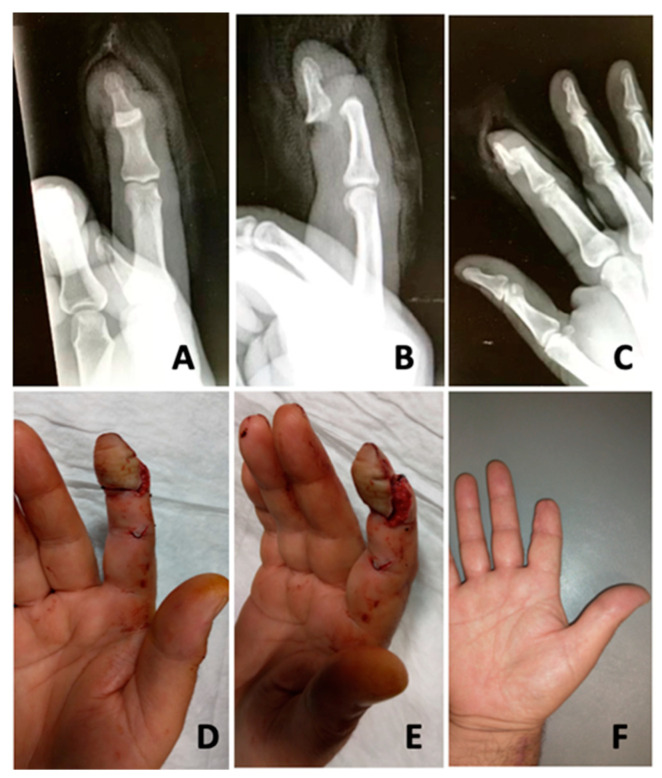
Avulsion injury of the distal phalanx of the second finger. (A,B) Volar and lateral views of the tissue continuity shown only by the flexor tendon. (C–E) Frontal, oblique and lateral radiographs showing complete volar dislocation of the distal phalanx without fracture. (F) Final aspect after reduction.
